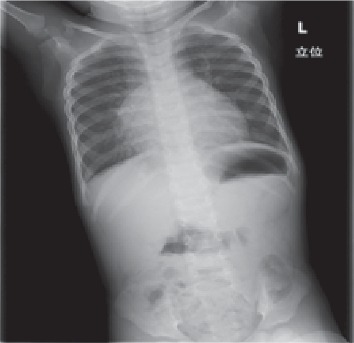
Posteroanterior chest radiograph showing reticular micronodular opacities bilaterally in the first visit.
